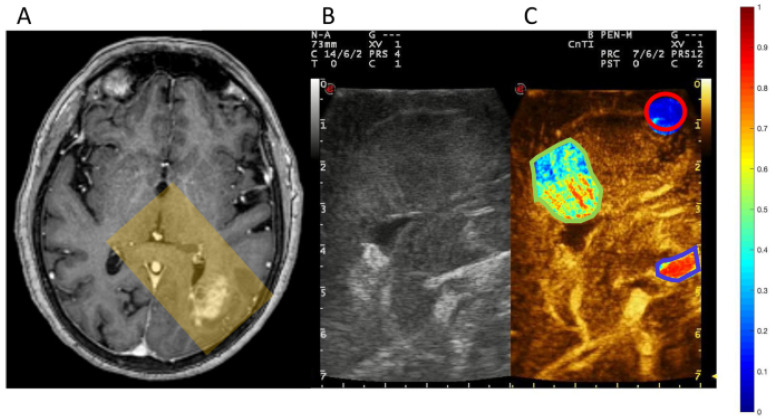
Qualitative assessment of microbubble concentration in specific brain areas for a case involving parietal glioblastoma: (A) Gadolinium-enhanced T1-weighted axial MR demonstrates the tumor. The translucent yellow rectangle highlights the insonation plane through the craniotomy, aligning with the ultrasound (US) images. US monitors screenshots of low mechanical index B-mode imaging (B) and contrast-enhanced ultrasound (CEUS) images (C). In (C), three regions are outlined in blue (artery), red (white matter), and green (tumor), demonstrating microbubble density using a JET color scale. Reprinted with permission from Prada et al. [91].
